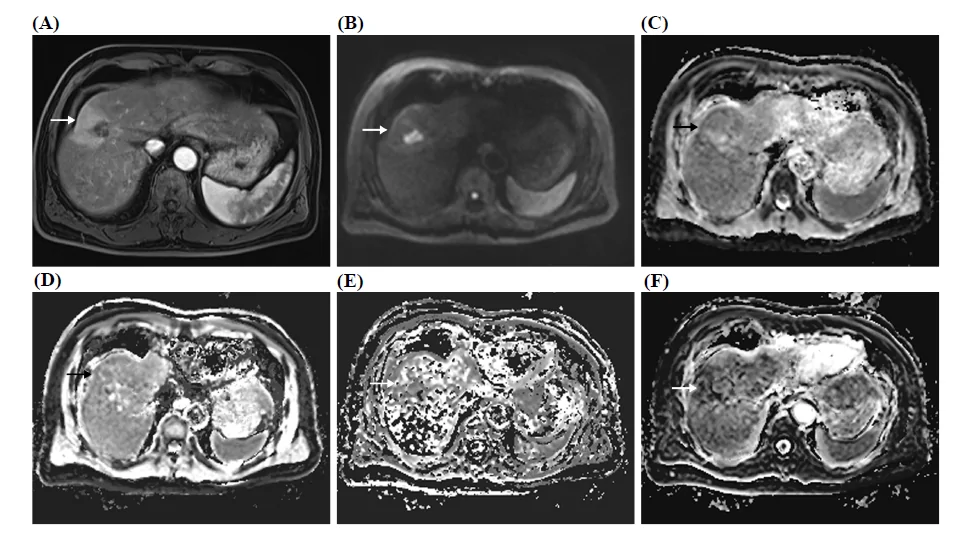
MR images in a 57-year-old man with an inflammatory reaction zone. Axial (A) enhanced fat-suppressed T1-weighted MR image shows the lesion with a peripheral rim of enhancement (arrow). Axial (B) DWI map shows a slightly higher heterogeneous signal intensity. Axial (C) ADC map shows a slightly lower heterogeneous signal intensity. Axial (D) * D* map shows a slightly lower heterogeneous signal intensity. Axial (E) * D map shows a heterogeneous isointense signal. The axial (F) f map shows a heterogeneous, isointense signal. The calculated mean values of ADC, *D, D, and *f* for the recurrent HCC were 1.13×10^-3^ mm^2^/s, 0.85×10^-3^ mm^2^/s, 38×10^-3^ mm^2^/s, and 21%, respectively.
